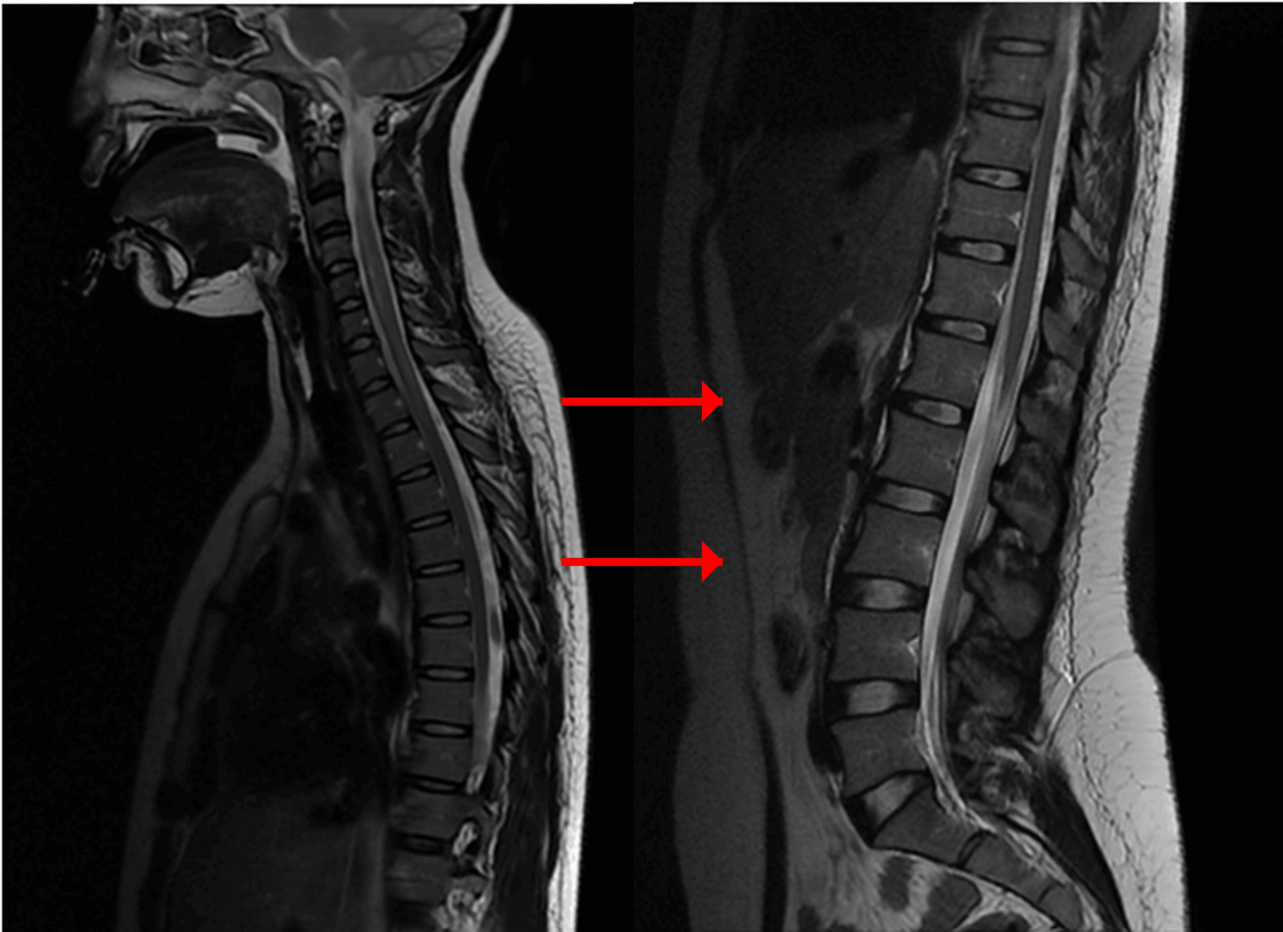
Sagittal STIR cervical spinal MRI confirming inflammatory lesion Sagittal STIR image confirming the inflammatory nature and longitudinal extent of the cervical intramedullary lesion (arrows). STIR: Short tau inversion recovery; MRI: Magnetic resonance imaging
